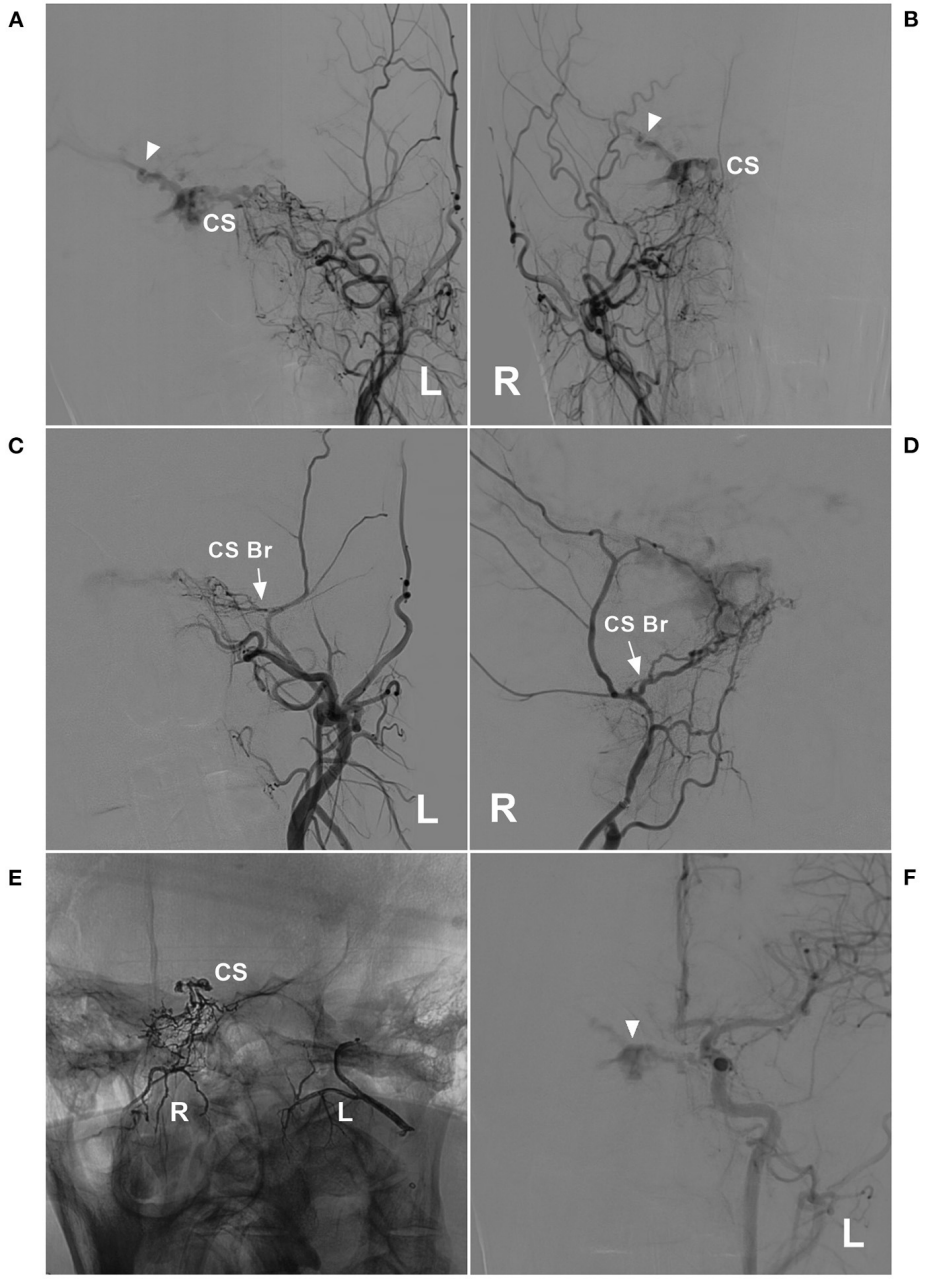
Unsuccessful type Ia EVT of a CS DAVF. (A,B): Left (A) and right (B) DSA of the anterior posterior view showed a CS DAVF with cortical vein drainage in the Sylvian fissure (triangles). (C,D) Left (C) and right (D) DSA showed the CS branch supplying the DAVF (arrows). (E) X-ray film showed bilateral embolization with Onyx. Onyx was successfully injected into the CS. (F) DSA of the left carotid artery showed that the EVT was incomplete, and the DAVF remained visible (triangle). Br, branch; CS, cavernous sinus; DAVF, dural arteriovenous fistula; DSA, digital subtraction angiography; EVT, endovascular treatment; L, left; R, right.
